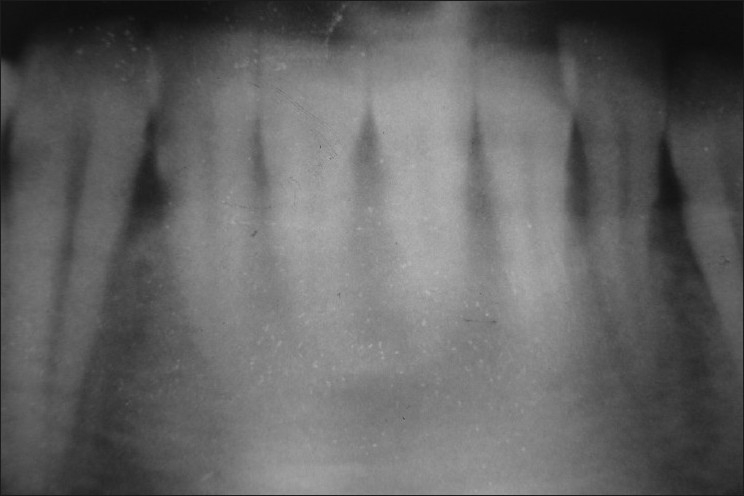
Closer view of orthopantomograph of the subject showing5 mandibular incisors
